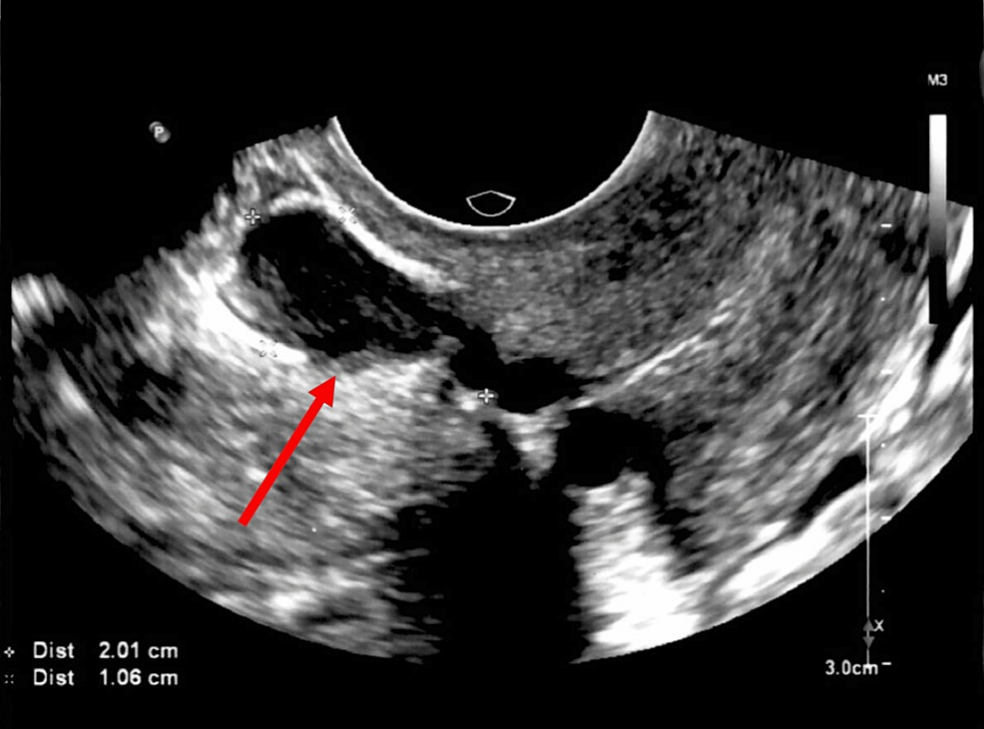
Transvaginal ultrasound showing cesarean scar defect with seroma and scar endometriosis
